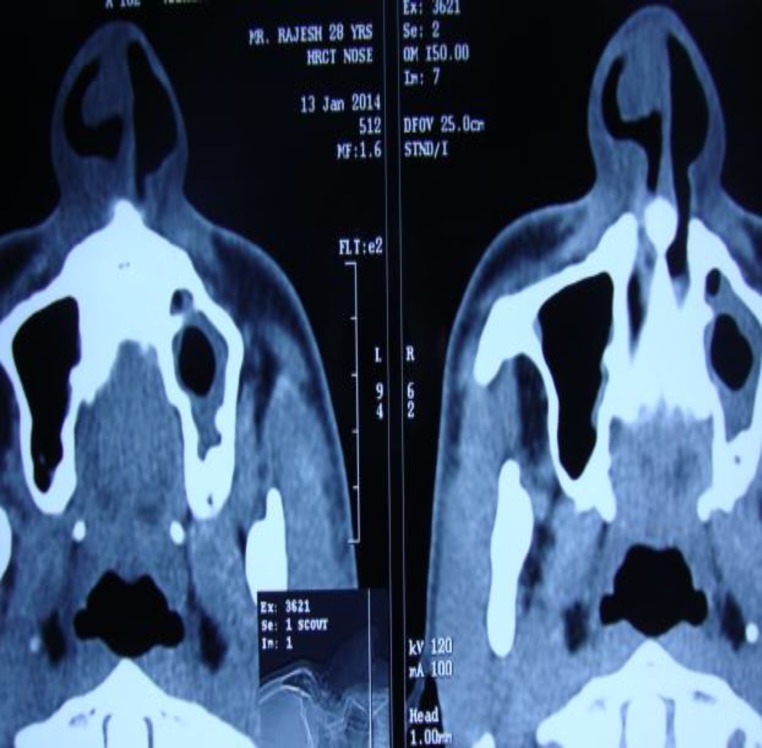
CT Scan (Axial section) shows a mass arising from the septum
